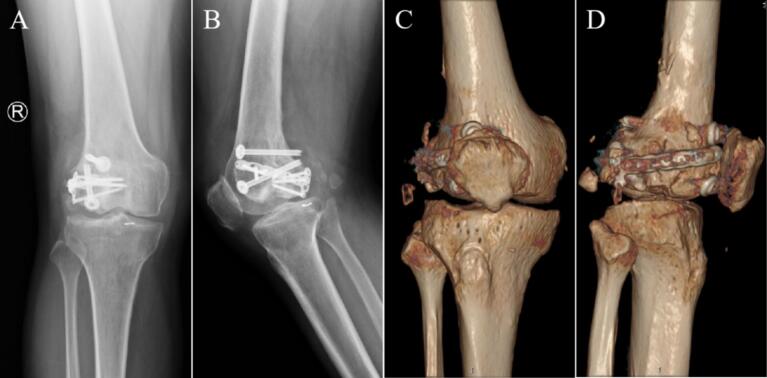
The preoperative images at our hospital. (A) AP view of the right knee X-ray. (B) X-ray lateral view of the right knee. (C) CT reconstruction AP view of the right knee. (D) three-dimensional CT reconstruction lateral view of the right knee.
